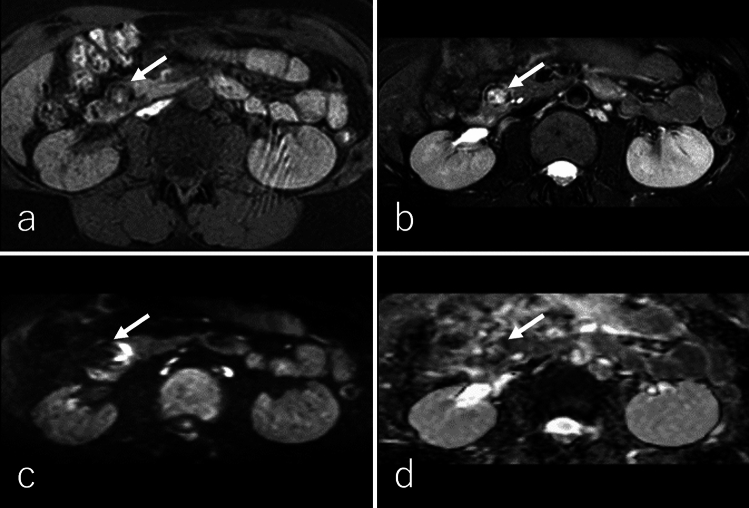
A 43-year-old male patient with a solid-pseudopapillary neoplasm. a Fat-suppressed T1-weighted imaging (FS-T1WI), b fat-suppressed T2-weighted imaging (FS-T2WI), c diffusion-weighted imaging (DWI) with *b* value of 800 s/mm^2^, and d an apparent diffusion coefficient (ADC) map. The tumor is located in the pancreatic head (arrow), measuring 2.0 cm in diameter, and exhibits expansile growth with well-defined margins. The central region of the tumor exhibits heterogeneous hyperintensity on FS-T1WI, FS-T2WI, and DWI, with an equivalent ADC values (1.46 × 10⁻^3^ mm^2^/s) relative to the pancreatic parenchyma. Additionally, the tumor demonstrates a hypointense rim on FS-T1WI, FS-T2WI, and DWI, corresponding to the peripheral rim calcification observed on CT (shown in Fig. 13)
